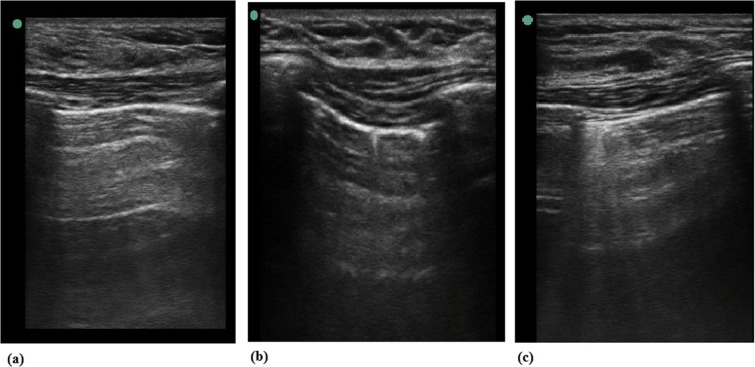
Transthoracic lung ultrasound (LUS) score image (a) Modified lung ultrasound scores 0, (b) Modified lung ultrasound scores 1, (c) Modified lung ultrasound scores 2.
